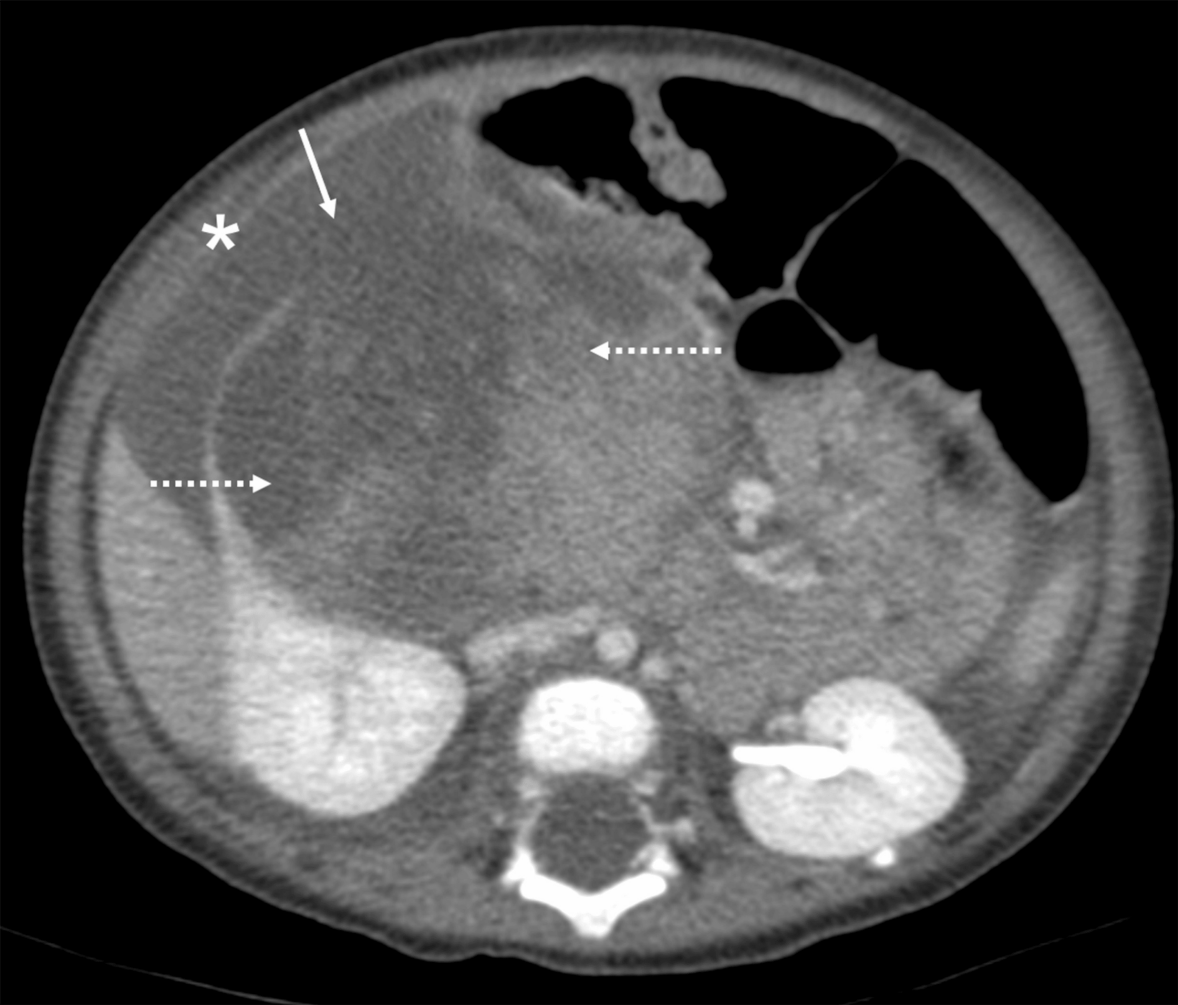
The axial CT shows WT in the right kidney (dashed arrows) with evidence of tumor spillage seen as discontinuous tumor edge (arrow) and ascites (*). CT: computed tomography; WT: Wilms tumor
